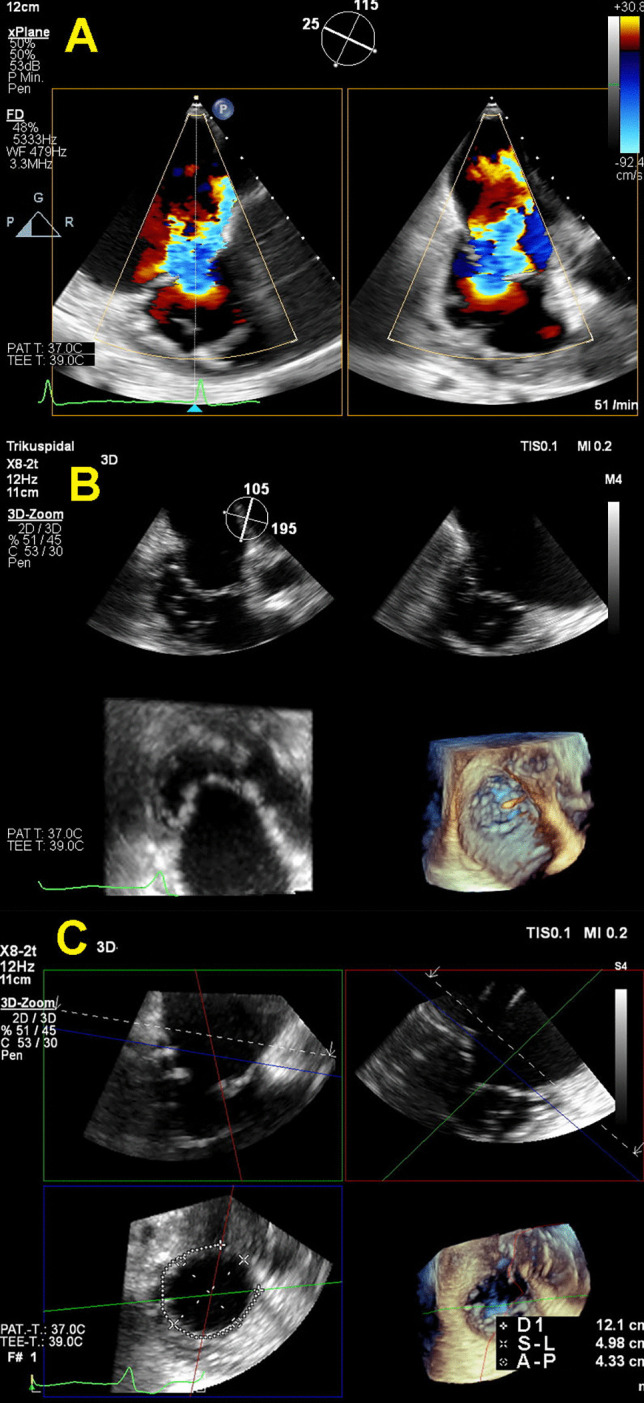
Patient with severe FTR before interventional annuloplasty (Panel A), 3D echocardiographic evaluation of tricuspid valve and annulus (Panels B and C). Footnotes: D1—perimeter of tricuspid annulus between aorta and coronary sinus, A-P—antero-posterior diameter of tricuspid annulus, S-L—septo-lateral diameter of tricuspid annulus
